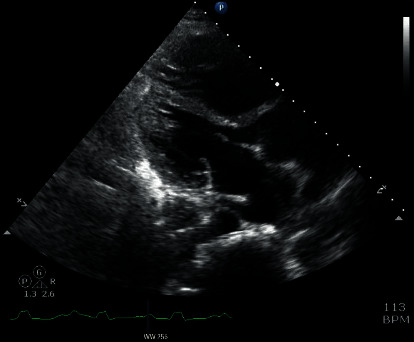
Transthoracic echocardiogram (TTE) demonstrating right sided ventricular hypertrophy.
